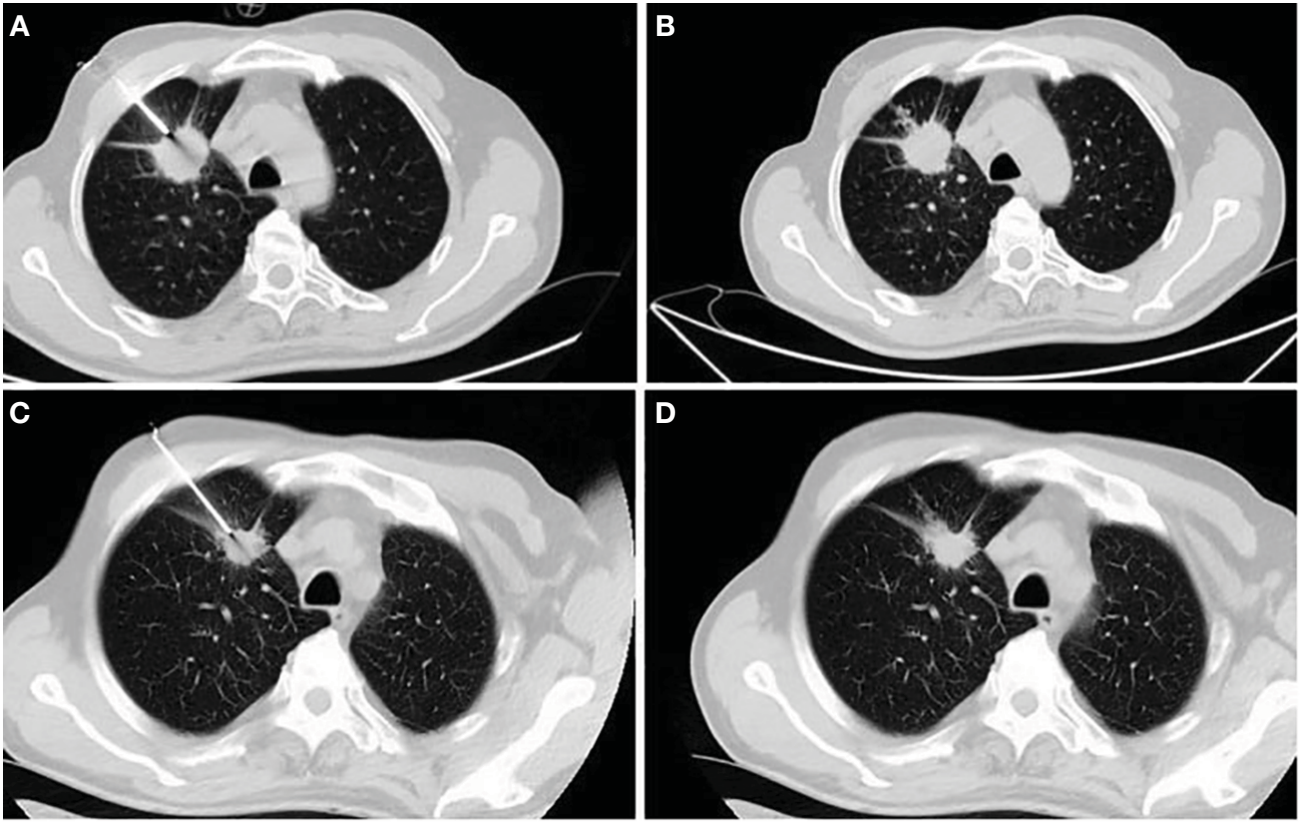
A 62-year-old man receiving his first biopsy (A, B) and rebiopsy at age 63 (C, D). (A) The needle enters the lesion through coaxial needles at first biopsy. (B) CT showed small amounts of bleeding along the needle path. (C) Disease progression after 10 months of gefitinib monotherapy and rebiopsy of the primary lesion using a coaxial biopsy needle. (D) There were no significant complications after rebiopsy.
